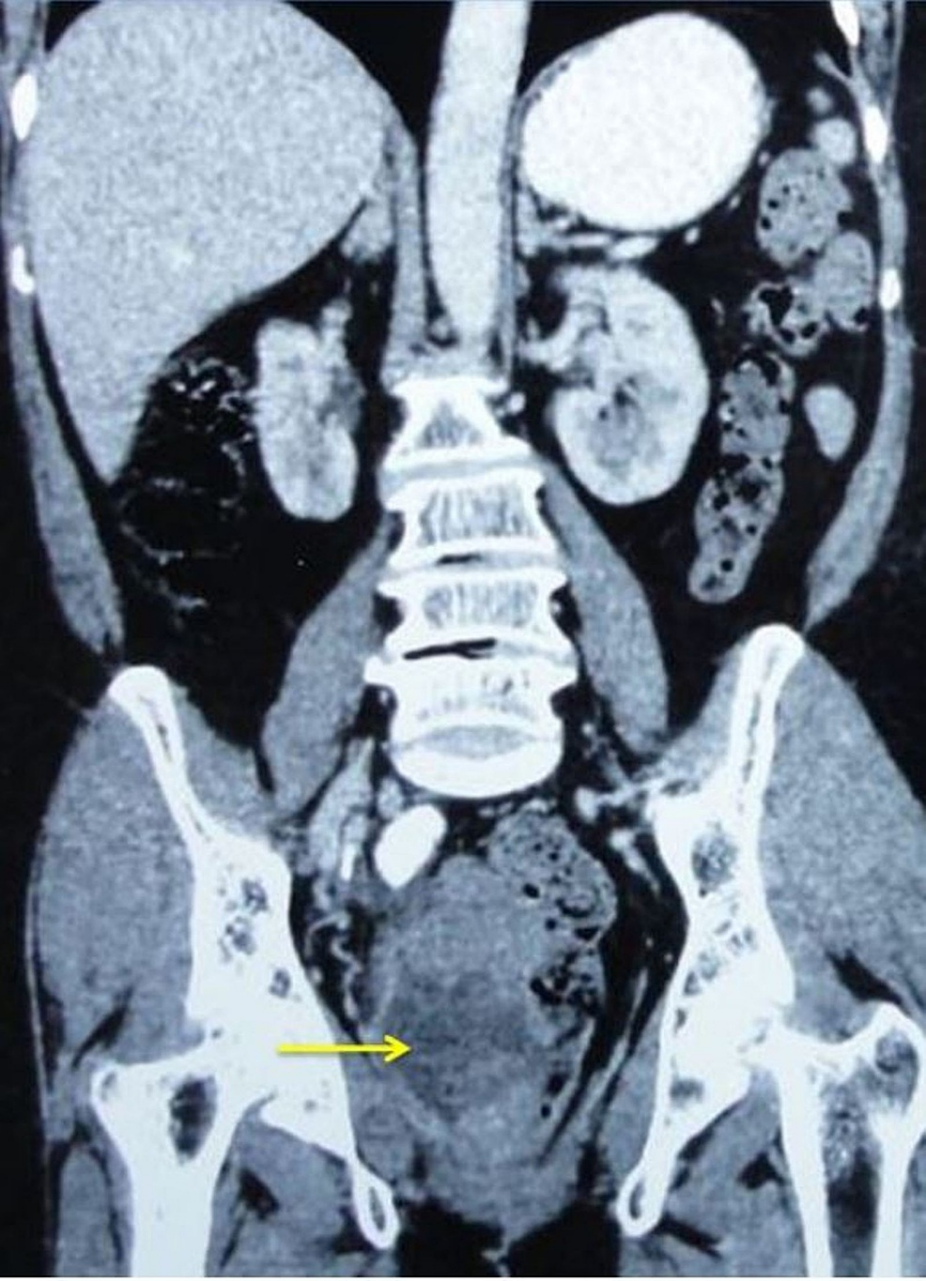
Computed tomography showing growth in the rectal wall (arrow), predominantly exophytic, causing thinning of the mesorectum, without any evidence of intra-abdominal metastasis.
